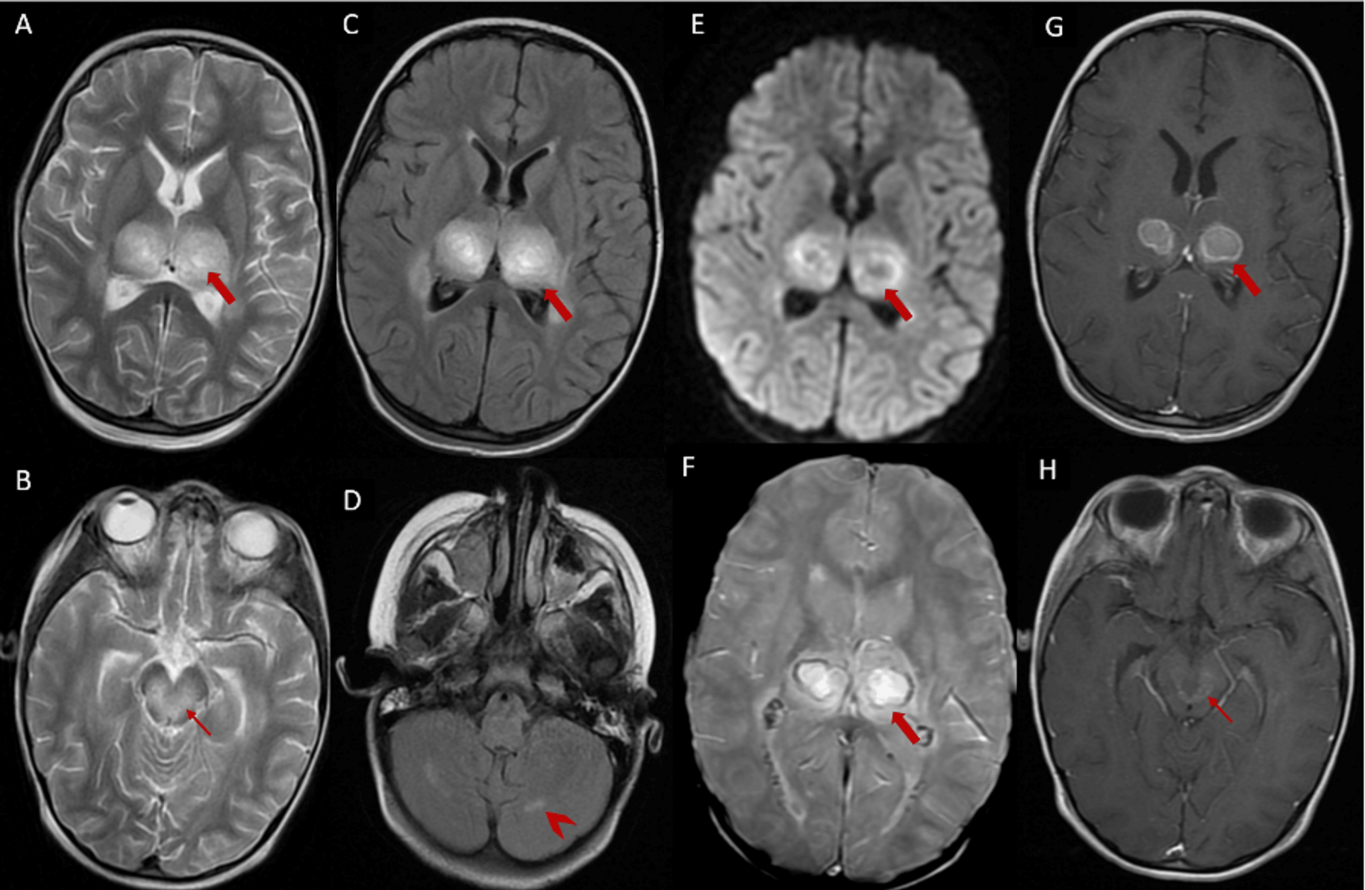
Axial multisequence MRI demonstrating necrotizing encephalopathy Axial T2-weighted images (A, B) and axial FLAIR images (C) show bithalamic lesions (thick arrows), involving the medial parts of the midbrain and brainstem tegmentum (thin arrow) and cerebellar white matter (arrowhead), with spontaneous hyperintensity on T2 and FLAIR, most pronounced at the thalami. Susceptibility-weighted imaging (F) shows a peripheral signal void consistent with hemorrhagic stigmata. Post-contrast axial T1-weighted images (G, H) show mild enhancement after contrast administration. The lesions are surrounded by a FLAIR hyperintense zone associated with peri-lesional edema.
